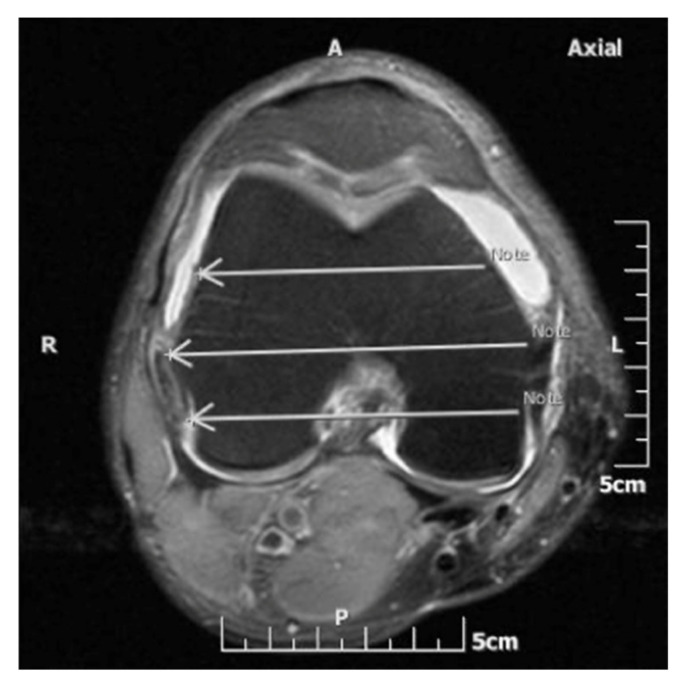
Axial fat-saturated proton density-weighted MR image at the level of the maximum mediolateral diameter of the femoral epicondyles. The middle plane (middle arrow) connects the medial and lateral femoral epicondyles. The anterior and posterior planes (anterior and posterior arrow, respectively) are drawn half way between the middle plane and a line tangent to the anterior and posterior femoral cortical margins, respectively.
